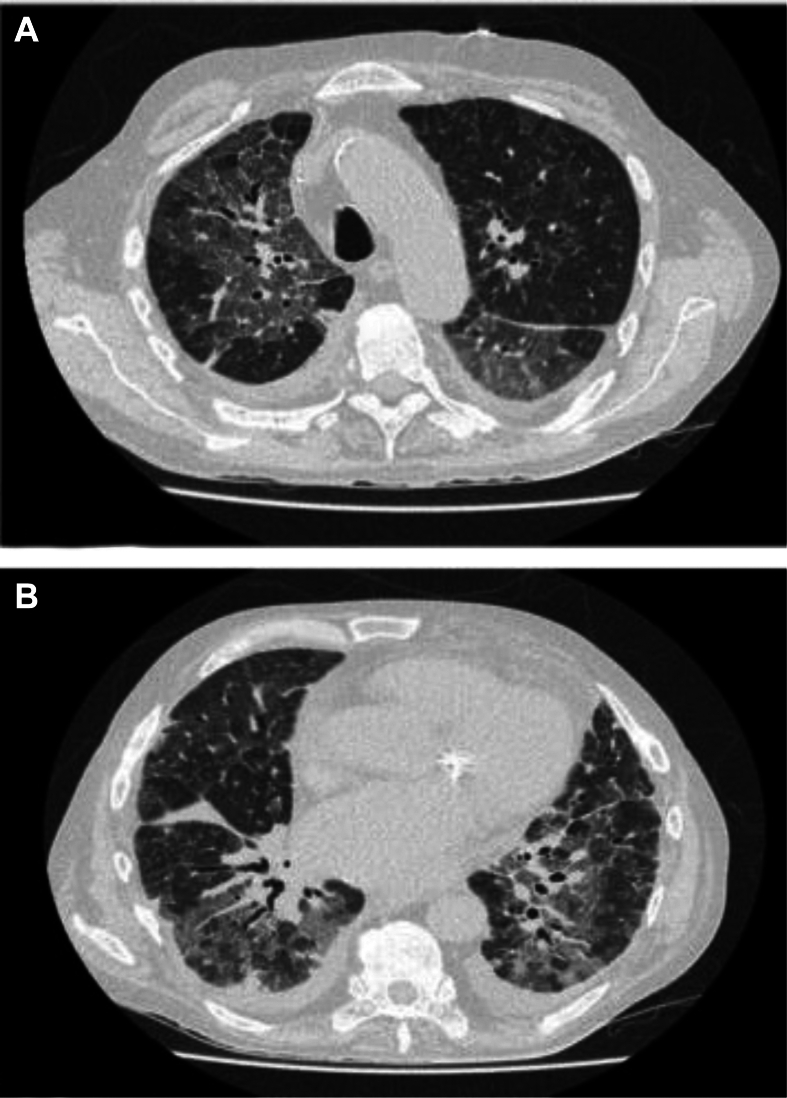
Chest computed tomography scan from Case 1 at the time of the episode of acute respiratory failure after immune checkpoint inhibitor initiation, showing diffuse interstitial lung opacities, with ground-glass opacities (A) and low-volume bilateral pleural effusion (B).
